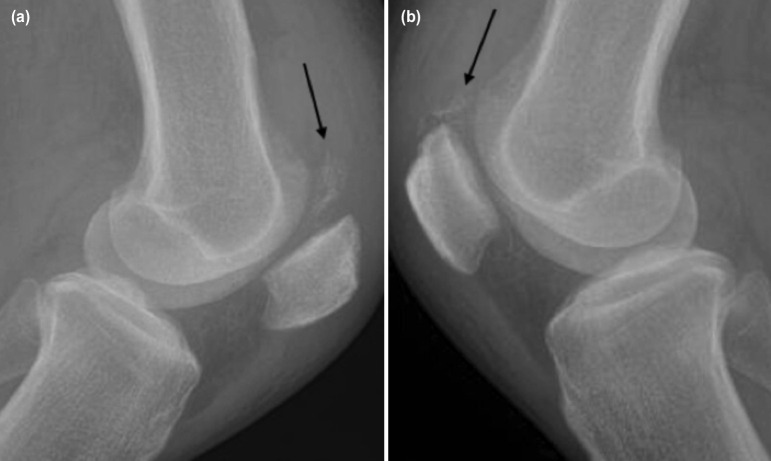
Lateral plain radiograph of the knee shows calcification (arrow) at the quadricep tendon insertion with patella baha, (a) right knee, (b) left knee.
